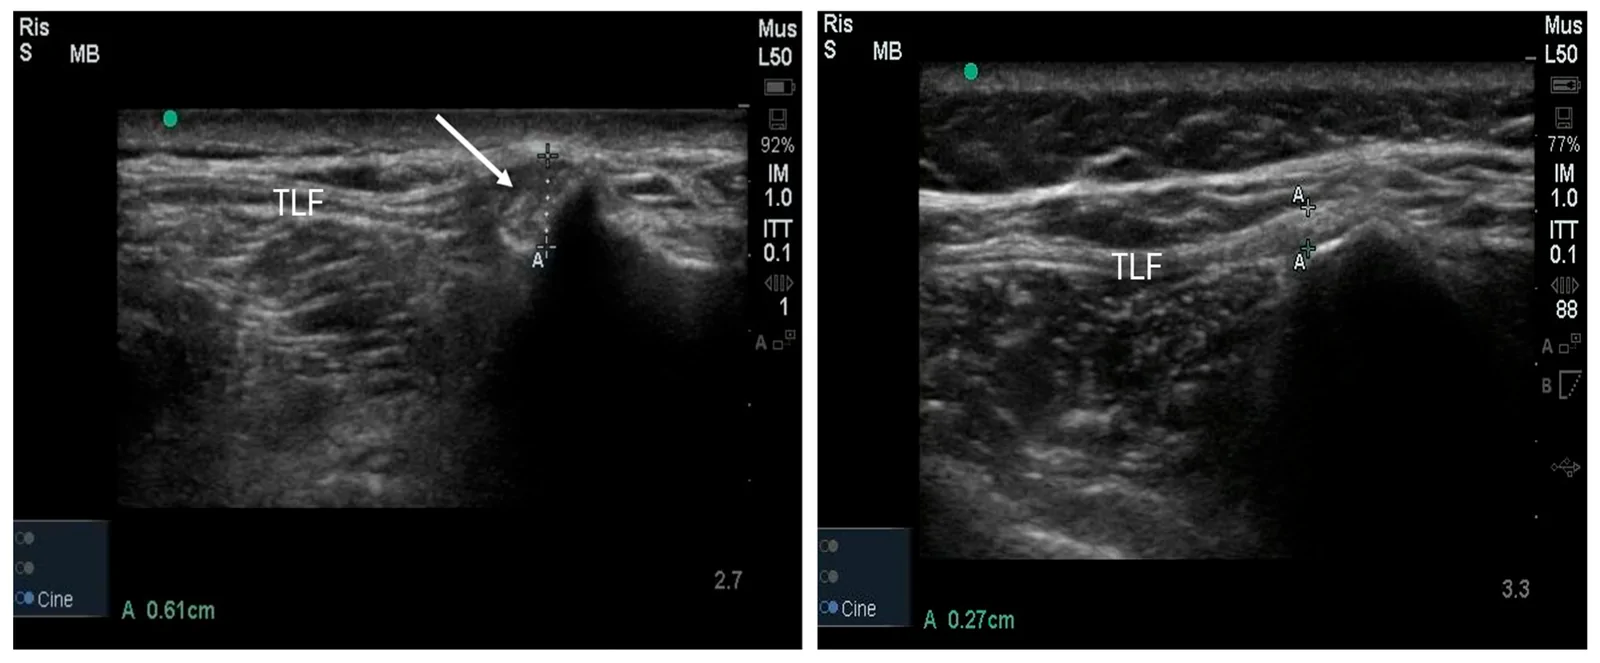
Ultrasound comparison of the thoracolumbar fascia (TLF) in short-axis view. On the left, pathological findings show evident thickening (white arrow) at the insertion site on the posterior iliac crest; on the right, normal TLF appearance in a healthy control without thickening. TLF: thoracolumbar fascia. The letter A represents the electronic calipers used to measure the thickness of the thoracolumbar fascia (TLF).
